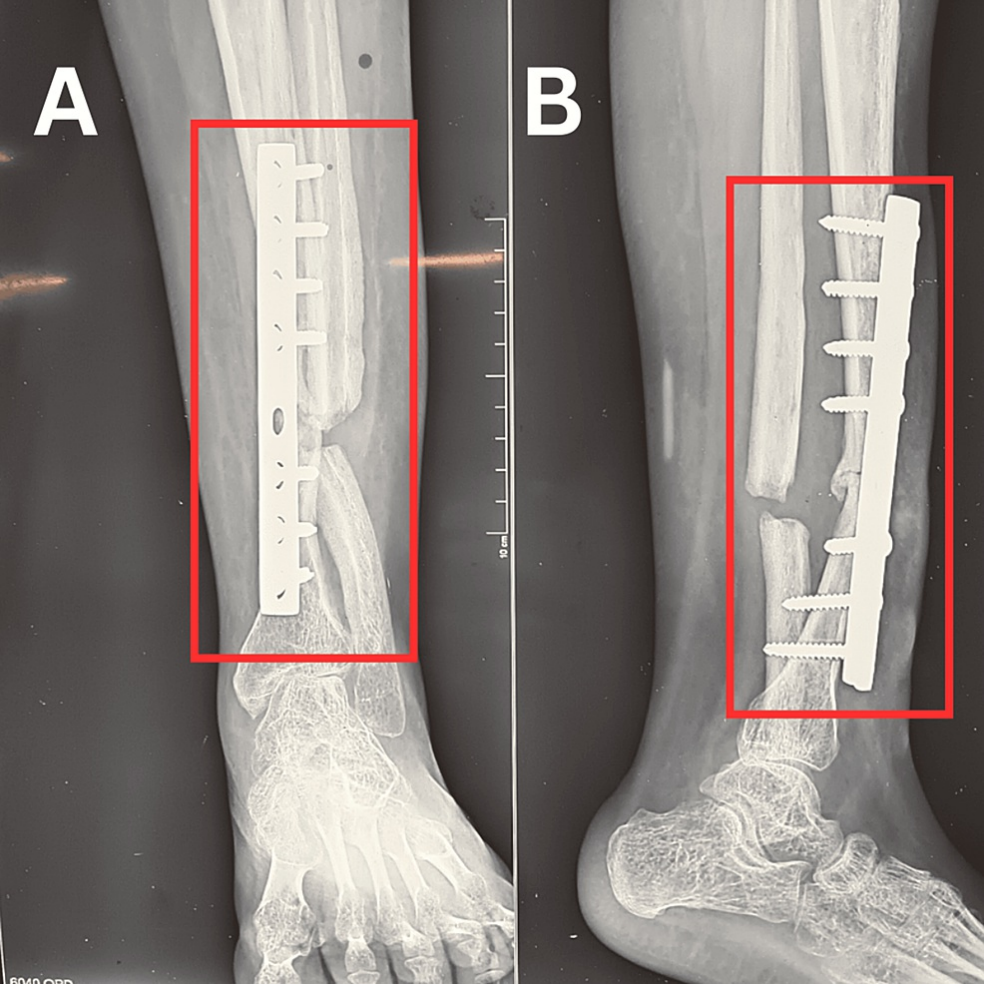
X-ray of right tibia with implants Rectangle represents open reduction internal fixation done by nailing and plating
